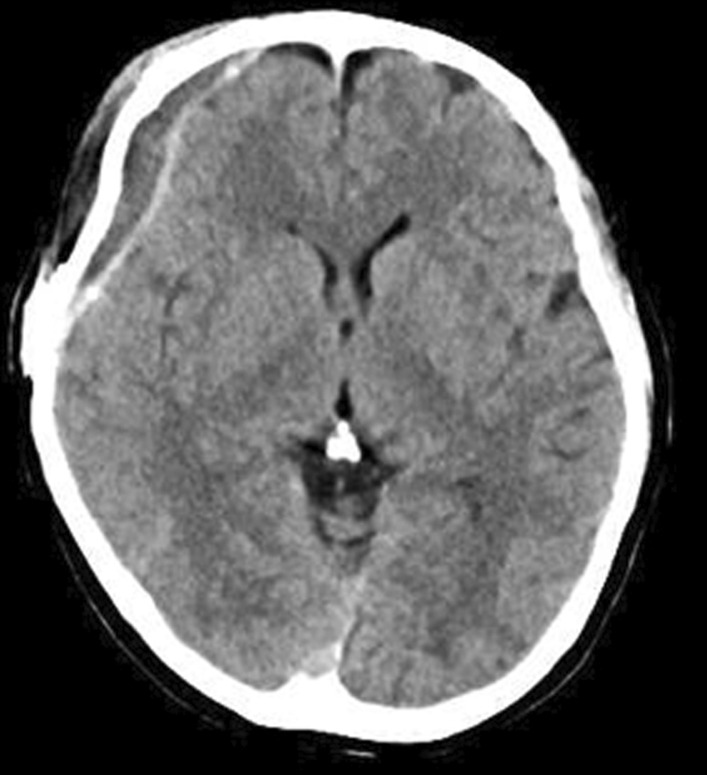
CT of first empyema. Isodense area of pooling was revealed in the right frontal epidural space
